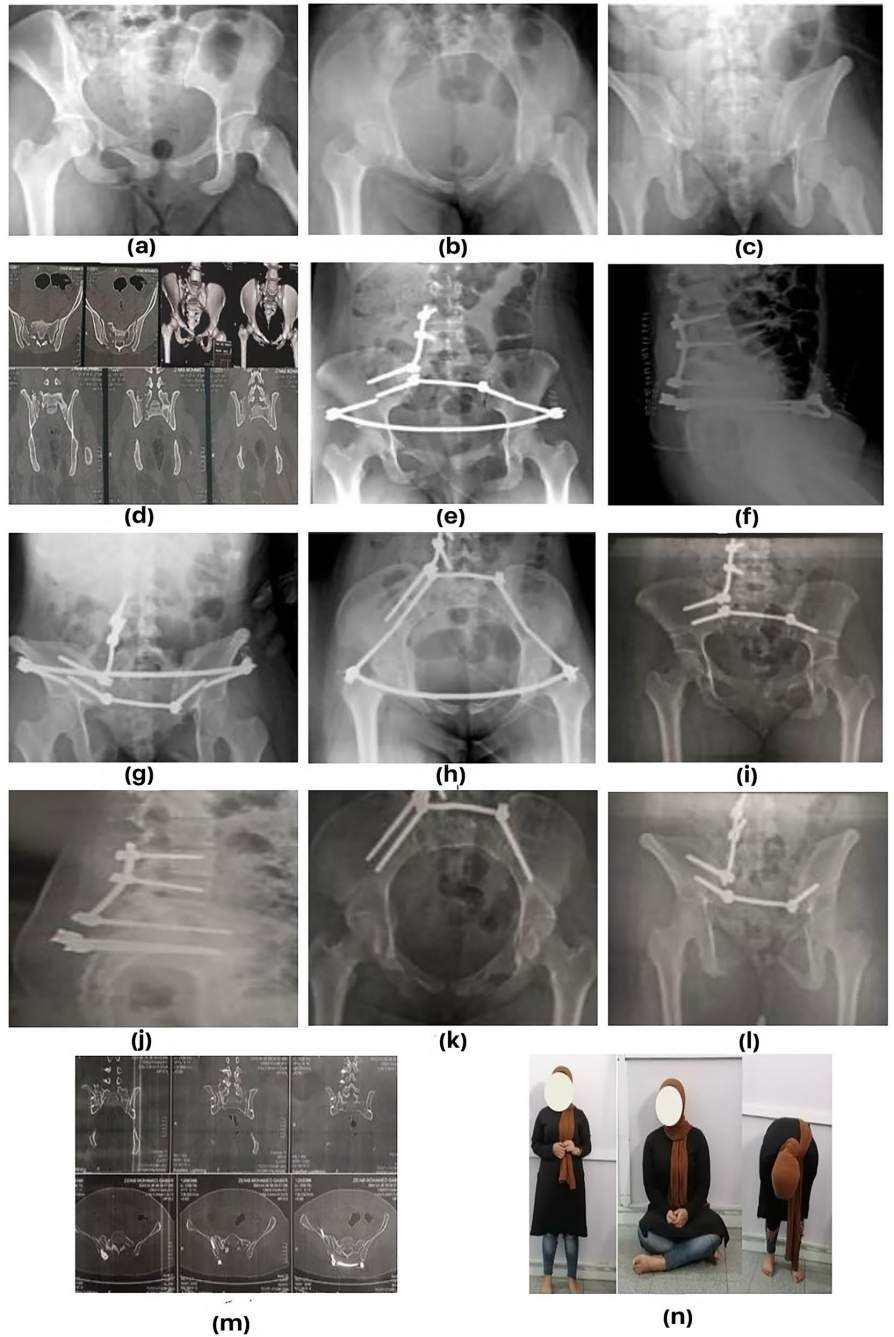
Preoperative (a, b, and c) radiographs of a 20-year female, (d) Computed tomography showing AO C1 sacral fracture, (e, f, g and h) Postoperative radiographs, (i, j, k and l) Plain X ray after 1 year showing removal of anterior infix and union of fracture, (m) CT showing fracture union, (n) Clinical and functional outcome after 12 months
